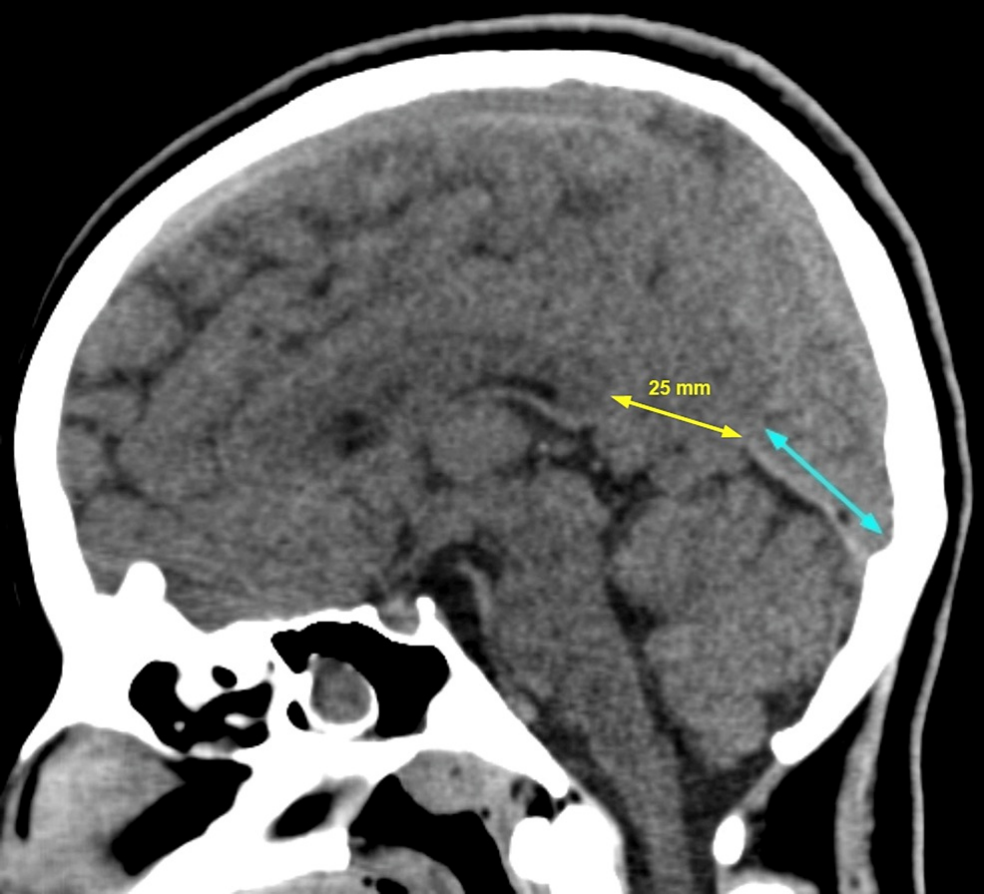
CT, brain window, left paramedian sagittal section. The cyan arrow shows the intact posterior part of the tentorium. Anterior to it is the defect (yellow arrow), allowing herniation of parts of the occipital lobe into the cistern below. CT: computed tomography
